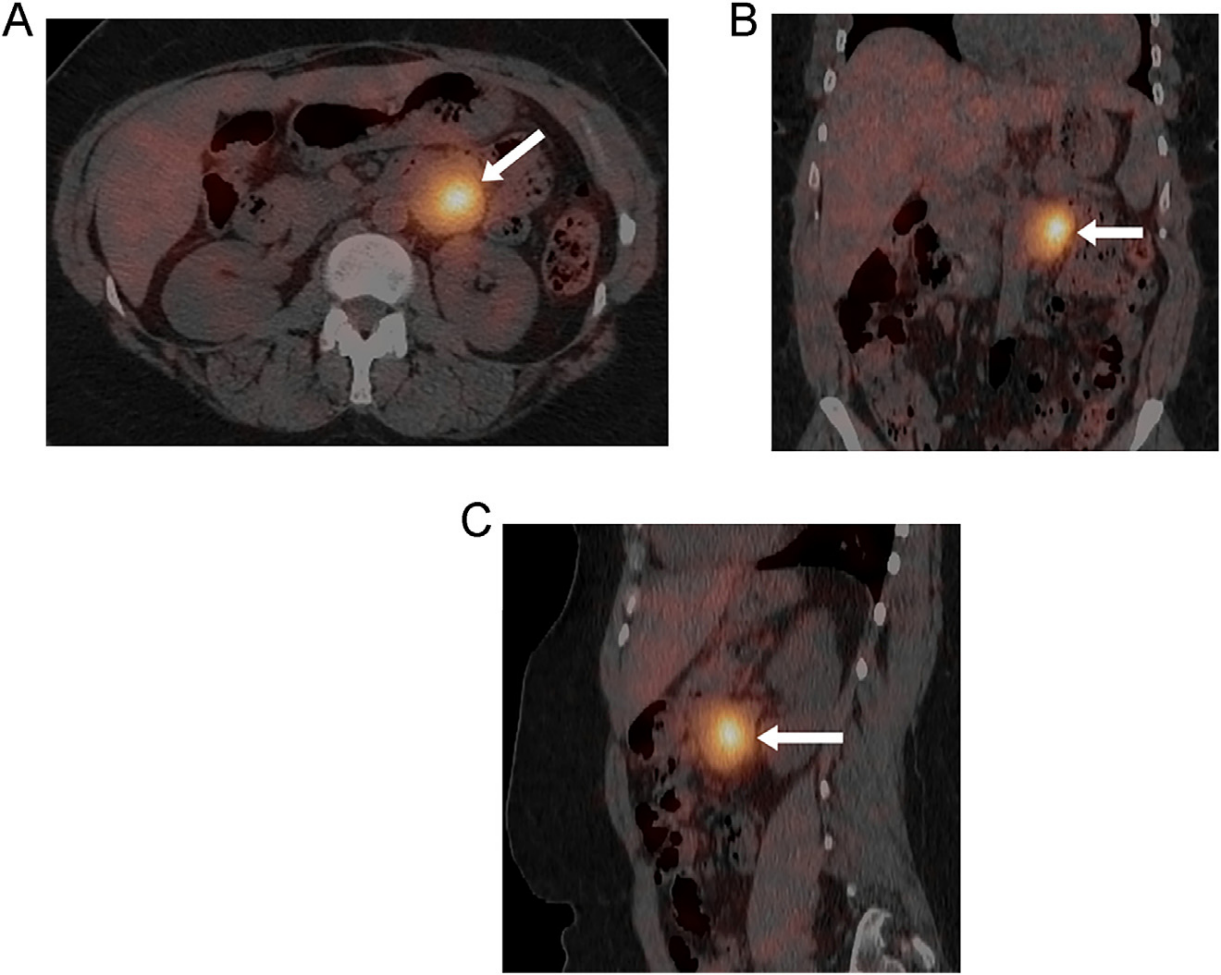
(A) Axial, (B) coronal, and (C) sagittal SPECT/CT images of the abdomen from an I-123 MIBG scan demonstrate radiotracer uptake in the left retroperitoneal lesion (arrows), confirming a neuroendocrine tumor. The uptake is most pronounced in the central to left aspect of the lesion, corresponding to the enhancing portion seen on the prior CT.
